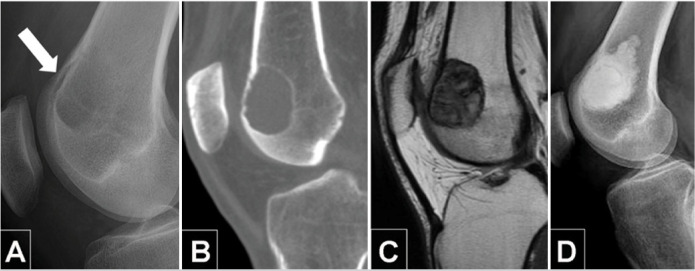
A) Preoperative radiograph and B) computed tomography scans displaying a meta-epiphyseal lesion causing endosteal erosion (Campanacci II) and expanding the anterior cortex of the distal femur (arrow). C) Contrast-enhanced MRI of the knee revealing the medullary and cortical boundaries of a giant cell tumor of bone (GCTB). D) Postoperative radiograph depicting the cavity filled with cement after curettage. Despite endosteal involvement and medullary osteolysis, joint structure and function were successfully restored.
